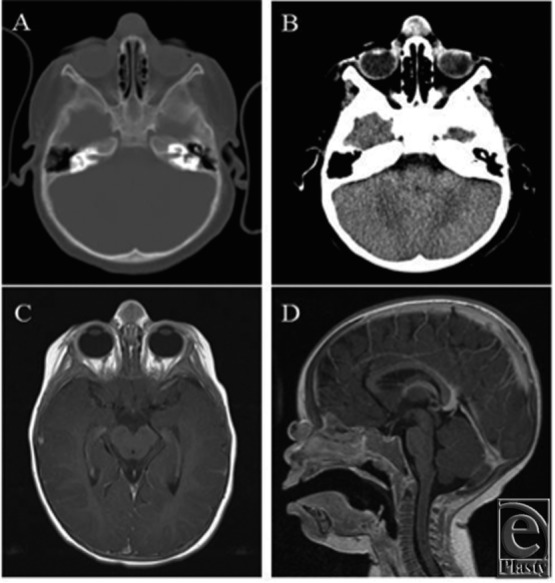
Diagnostic imaging: computed tomography of the head with contrast in the axial
